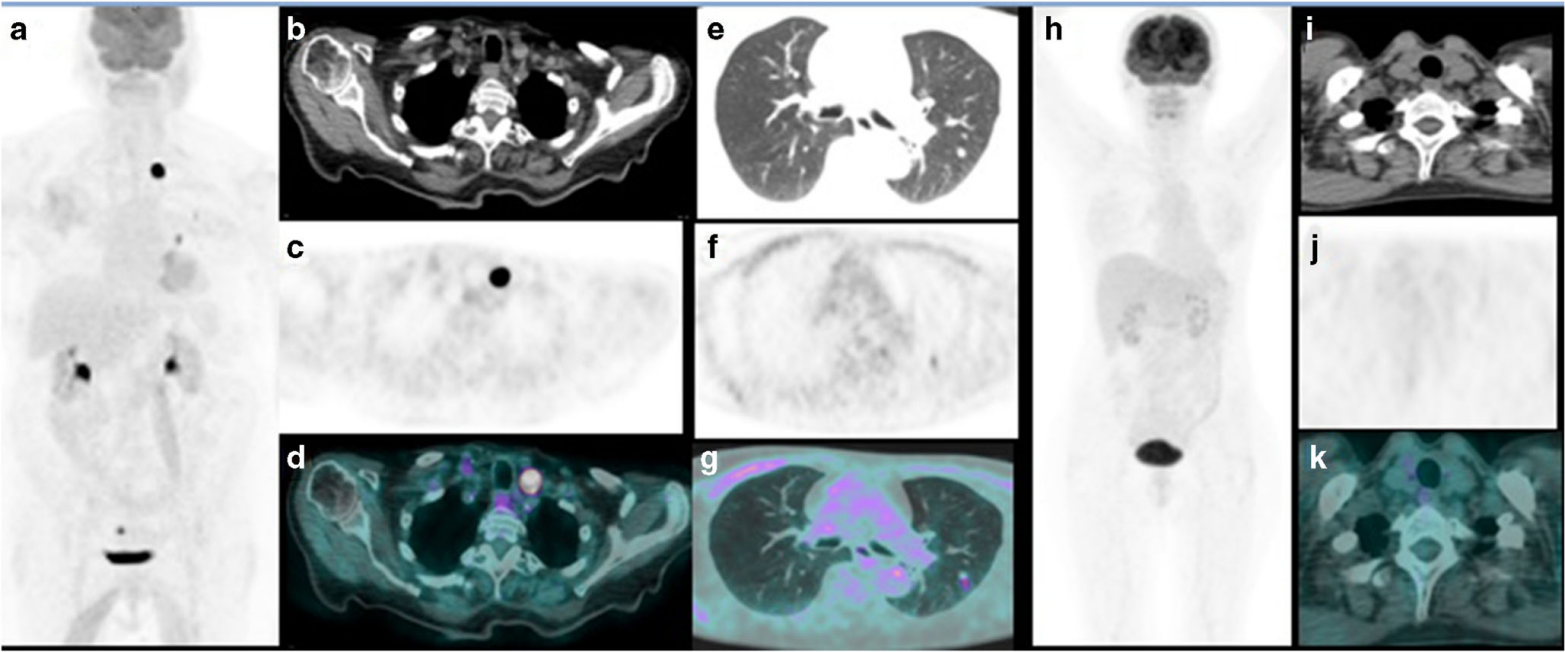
A representative case of a 71-year-old female with a conventional papillary carcinoma (pT3N1b according to AJCC 8th edition) who was imaged with [^18^F]FDG PET/CT while showing Tg of 6 ng/mL and TgDT of 1.12 years. Maximum intensity projection (MIP, a) showing several focal hypermetabolic lesions in the neck and chest. Axial CT (b), PET (c), and PET/CT (d) fused scan showing an increased FDG uptake in the left thyroid bed consistent of relapse. Thoracic CT (e), PET (f), and PET/CT (g) demonstrating a small lung nodule in the left superior lobe FDG-avid. Another case of a 49-year-old woman with follicular carcinoma (pT1bN0) with a negative [^18^F]FDG PET/CT (h). Neck CT (i), PET (j), and PET/CT (k) fused images showing no hypermetabolic lesions and Tg of 6 ng/mL but TgDT of 7.64 years
